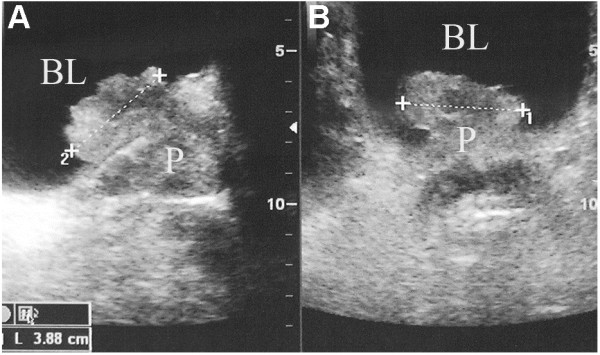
Abdominal color Doppler ultrasound showing hypoechoic region in the bladder neck and trigone with mucosal thickening in the bladder neck, interpreted as a bladder-occupying lesion. (A) sagittal plane; (B) transverse plane. P: prostate; BL: bladder.
